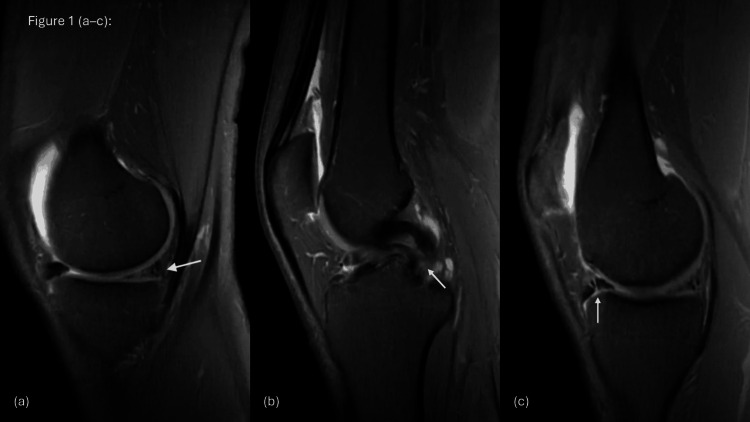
Sagittal T2-weighted MRI showing (a) the ghost sign (white arrow), (b) the double posterior cruciate ligament (PCL) sign (white arrow), and (c) a displaced anterior horn fragment (white arrow).
